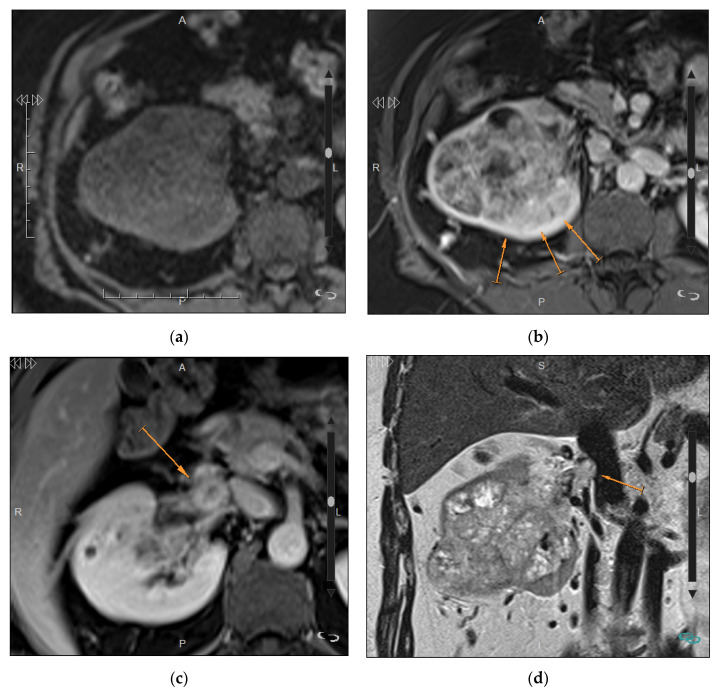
Expansive and infiltrative clear cell renal cell carcinoma in a 70-year-old man. (a) Axial pre-contrast T1-weighted image and (b) axial post-contrast T1-weighted image show a right renal mass with poorly defined margins between the mass and the adjacent renal parenchyma (arrows) with heterogeneous enhancement; (c) Axial postcontrast T1-weighted image also identifies a thrombus within the renal vein (arrow); (d) Coronal T2 weighted-image shows the heterogeneous renal mass involving the renal cortex and sinus, and confirms the thrombosis of the renal vein minimally bulging into the inferior vena cava, but without invading it (arrow). Scale size: 8 cm.
